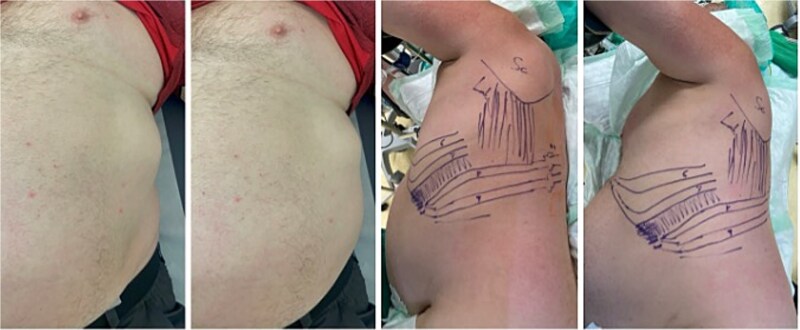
Preoperative images of the patient in the chest wall outpatient clinic (left) presenting with persistent deep thoracic back pain, mechanical ‘clicking’, and an intermittent bulge at the left costal margin during coughing. The two right images show the mapping of the injury pattern after indication for surgery, positioning in lateral right position and intra-op ultrasound detecting of the non-unions of the posterior column of the eighth and ninth rib as well as the costal margin rupture anterolateral between the seventh and eighth rib.
